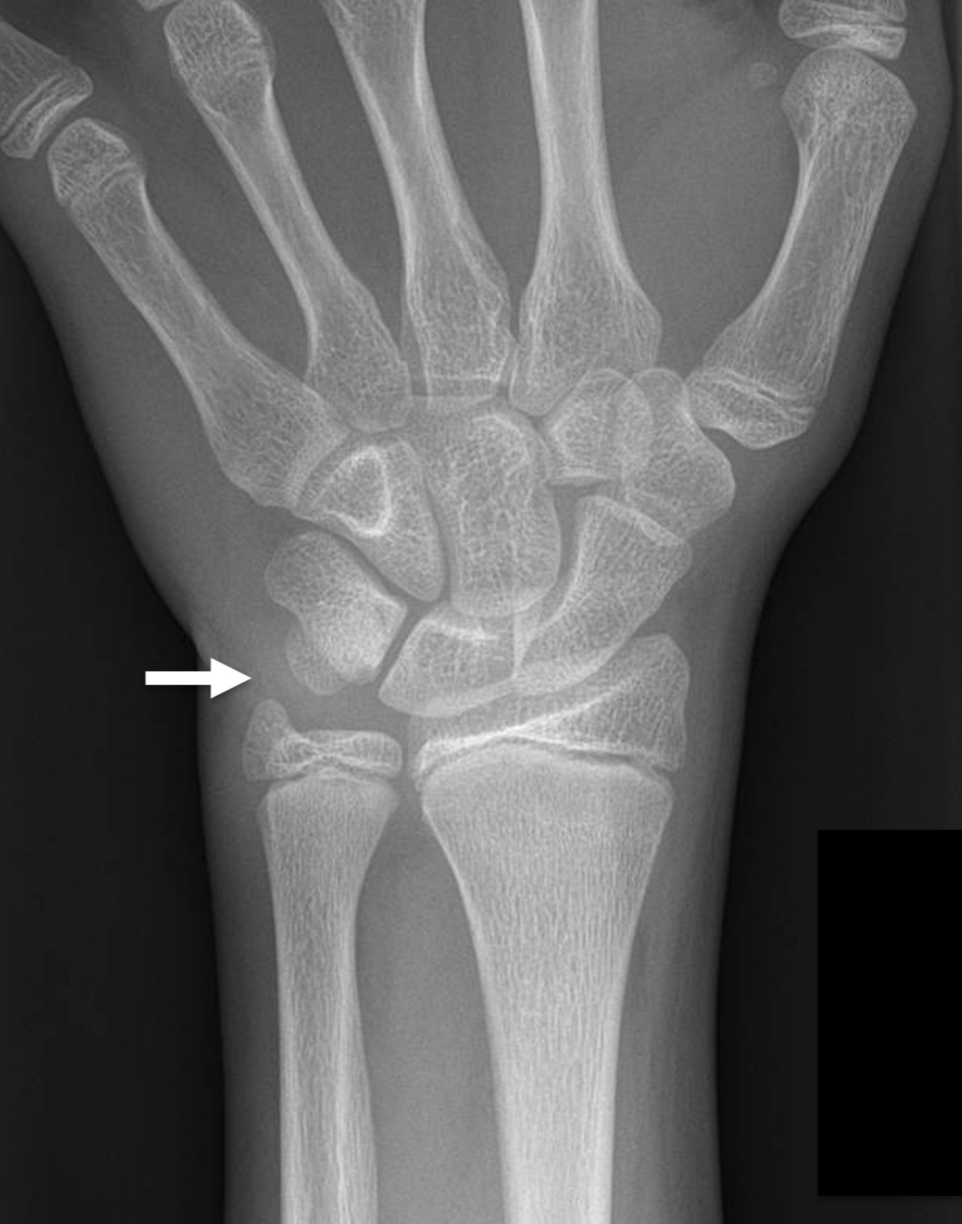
13-year-old boy with chronic ulnar sided left wrist pain This initial frontal radiograph of the left wrist was interpreted as normal. The arrow indicates the area of the patient's reported pain.
